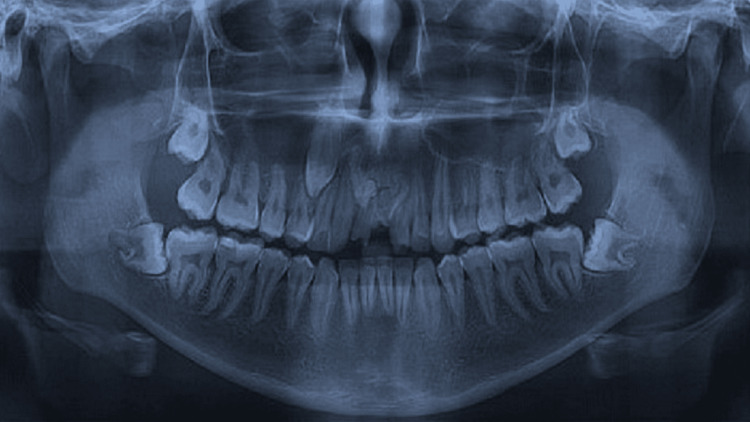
Orthopantomogram showing tooth-like structures in the anterior maxillary region.
